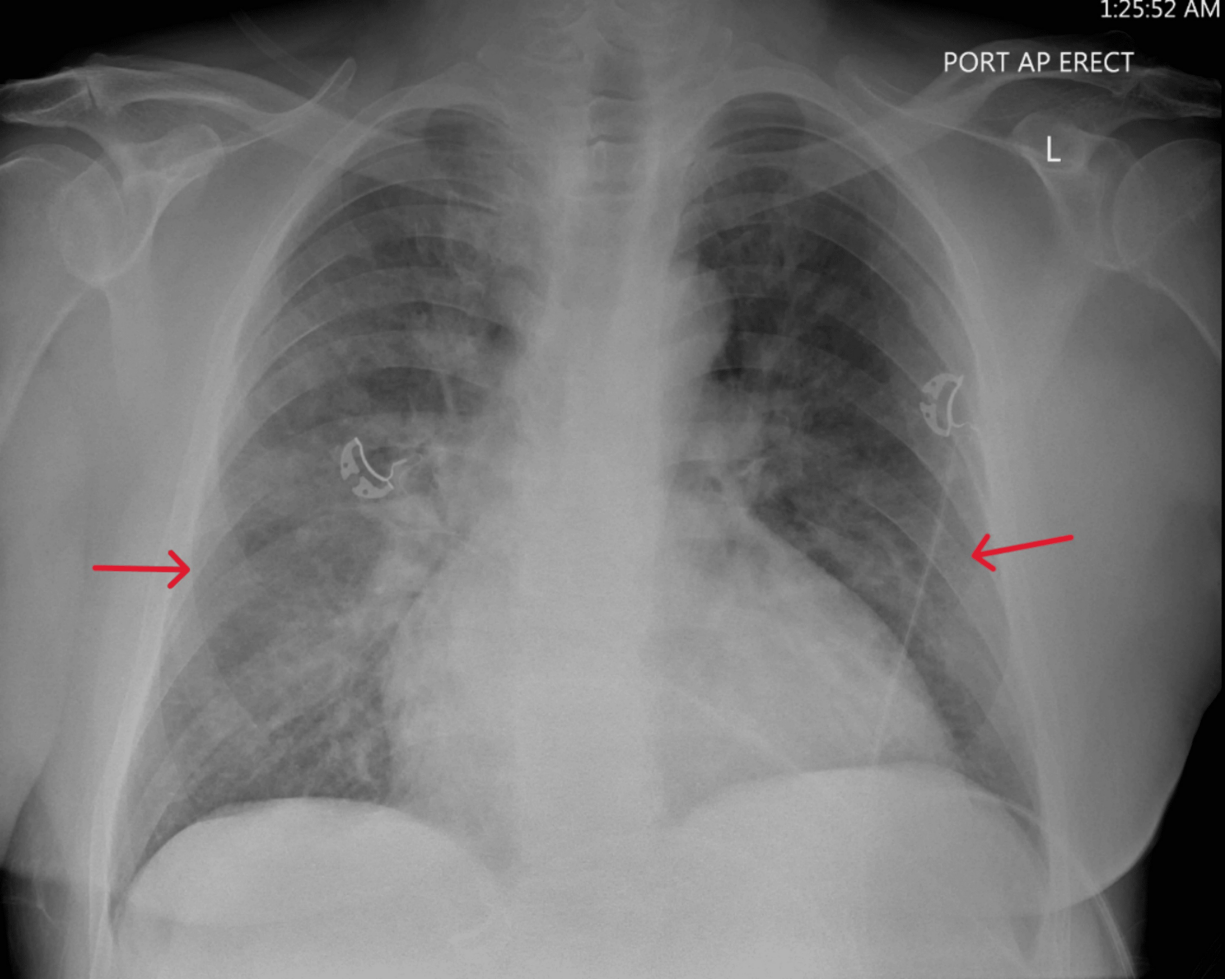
CXR (anteroposterior) demonstrates signs of pulmonary edema. Red arrows indicate increased bilateral opacities signifying pulmonary edema. CXR: chest x-ray
